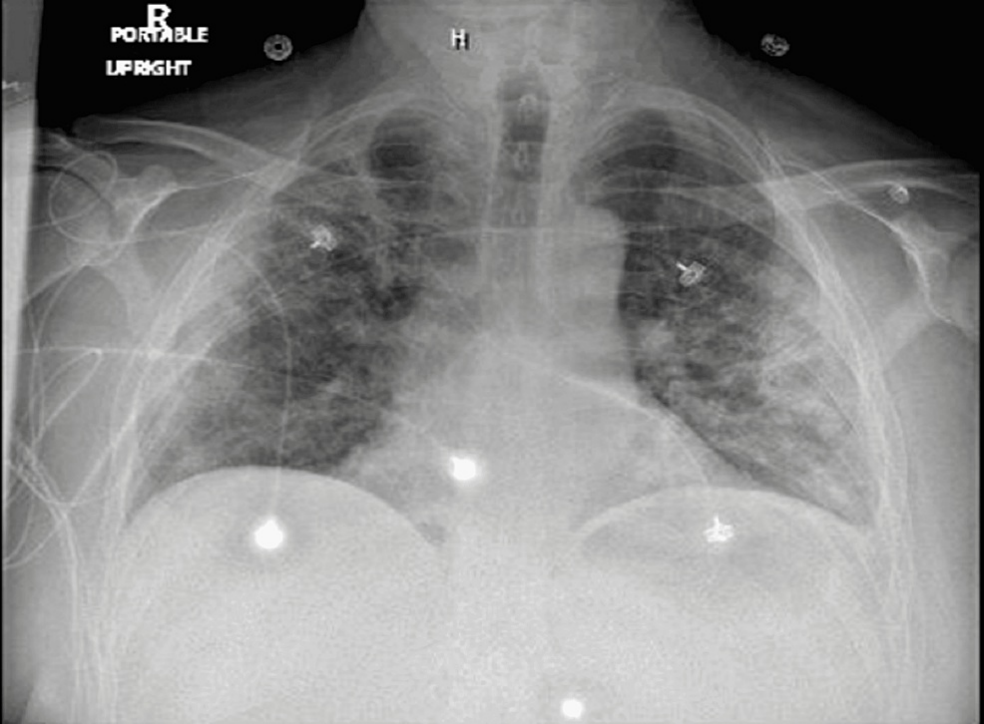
Chest x-ray showing patchy and confluent parenchymal infiltrates throughout the lungs.
